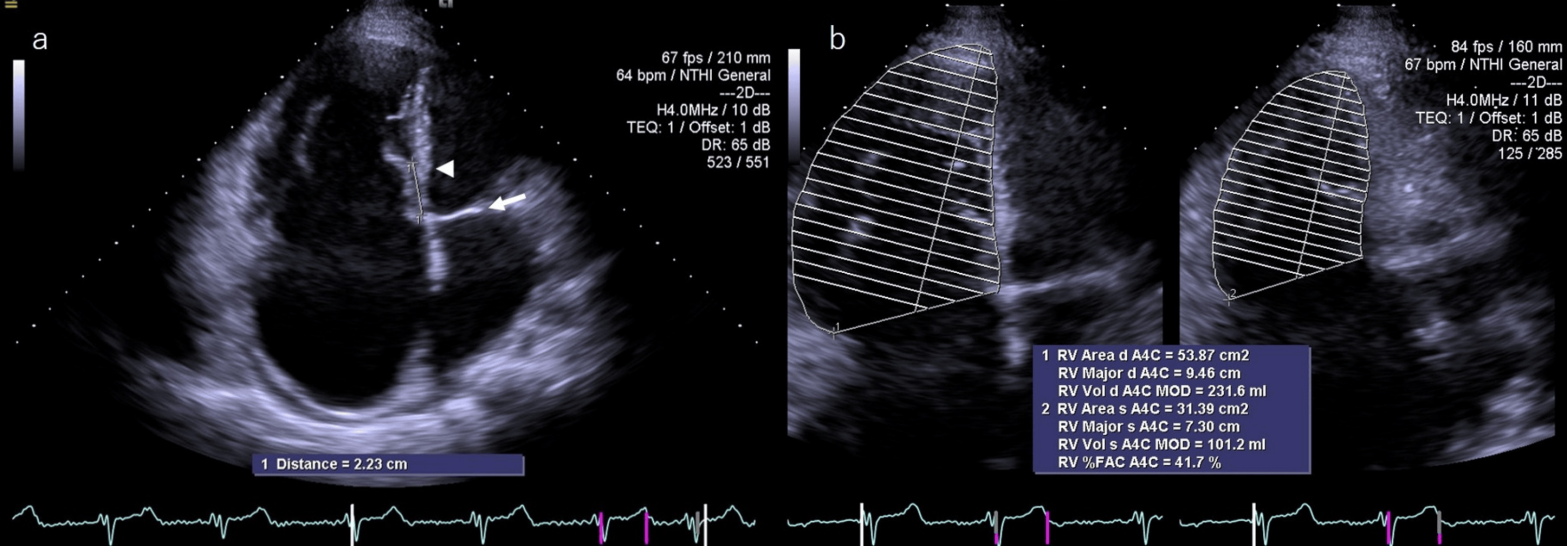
Preoperative transthoracic echocardiography in Case 1 (a) The septal leaflet of the tricuspid valve was displaced approximately 22 mm apically from the anterior mitral leaflet. The arrow marks the position of the anterior mitral leaflet, and the arrowhead indicates the location of the tricuspid septal leaflet. (b) Preoperative assessment of right ventricular function. RV Area d; Right ventricular end-diastolic area; A4C: apical four-chamber view; RV Major d: major axis diameter in diastole; RV Vol d: right ventricular end-diastolic volume; MOD: modified Simpson’s method; RV Area s: right ventricular end-systolic area; RV Major s: right ventricular major axis diameter in systole; RV Vol s: right ventricular end-systolic volume; RV %FAC: right ventricular % fractional area change
